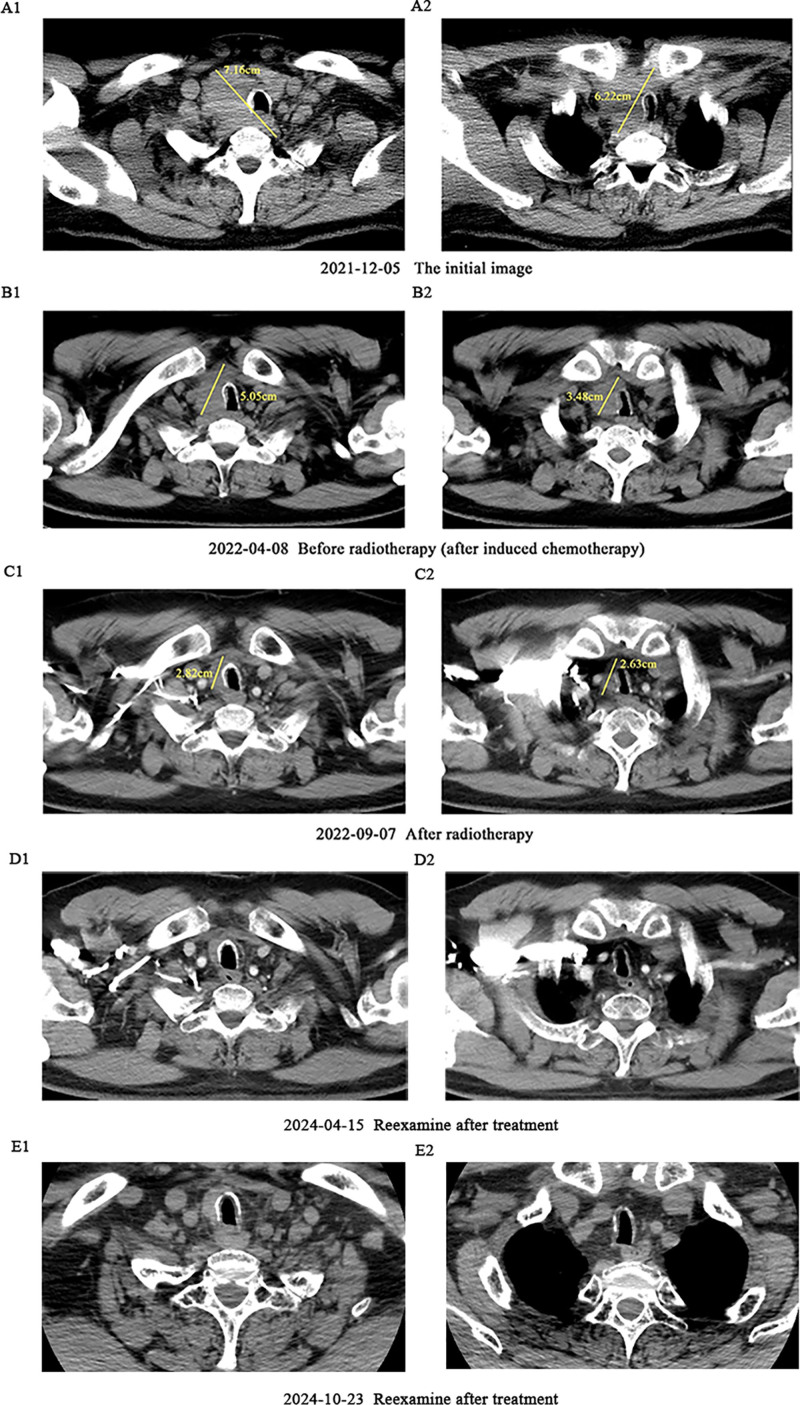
Depicts the CT image changes at the tumor level during the patient’s treatment. A1 and A2 are pre-treatment CT images; B1 and B2 are CT images after 5 cycles of chemotherapy and prior to concurrent radiotherapy; C1 and C2 are the first post-concurrent-radiotherapy review CT images; D1, D2, E1, and E2 are CT images taken at the post-treatment review. A1, B1, C1, D1, and E1 show the costal head of the first rib of the tumor, while A2, B2, C2, D2, and E2 represent the jugular notch level of the manubrium sterni of the tumor.
